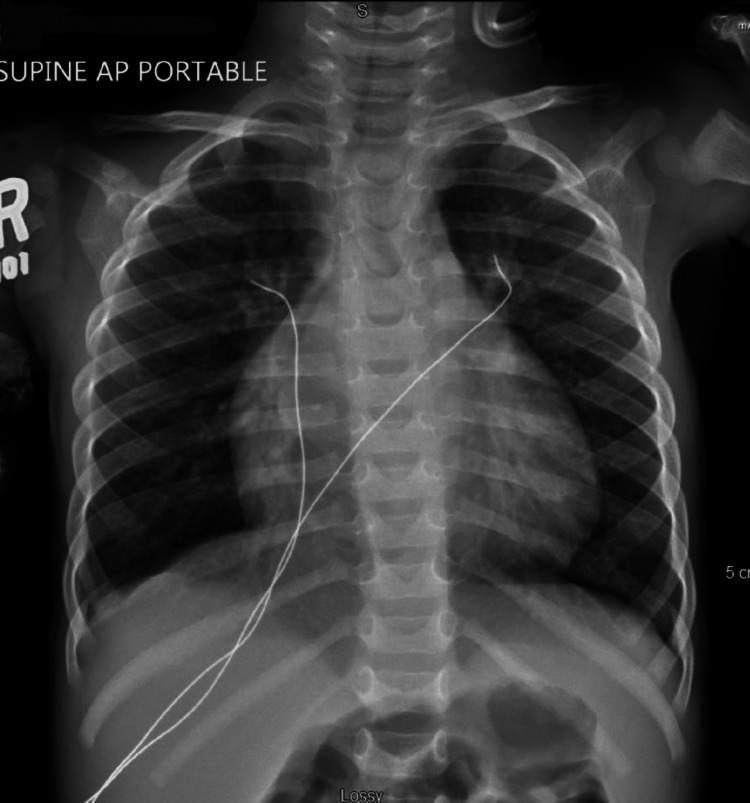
Chest radiograph for initial pediatric intensive care unit (PICU) admission
